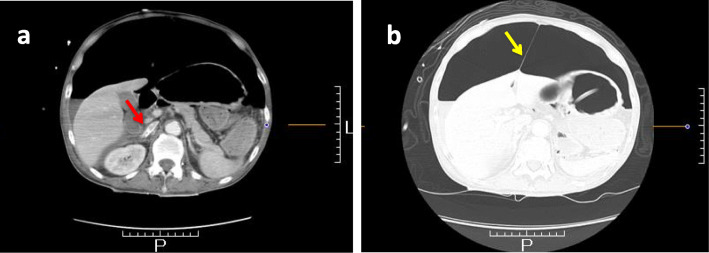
Contrast-enhanced computed tomography showing massive pneumoperitoneum. The inferior vena cava was collapsed (a, *red arrow*), and the round ligament of the liver was stretched owing to increased intra-abdominal pressure (b, *yellow arrow*)
